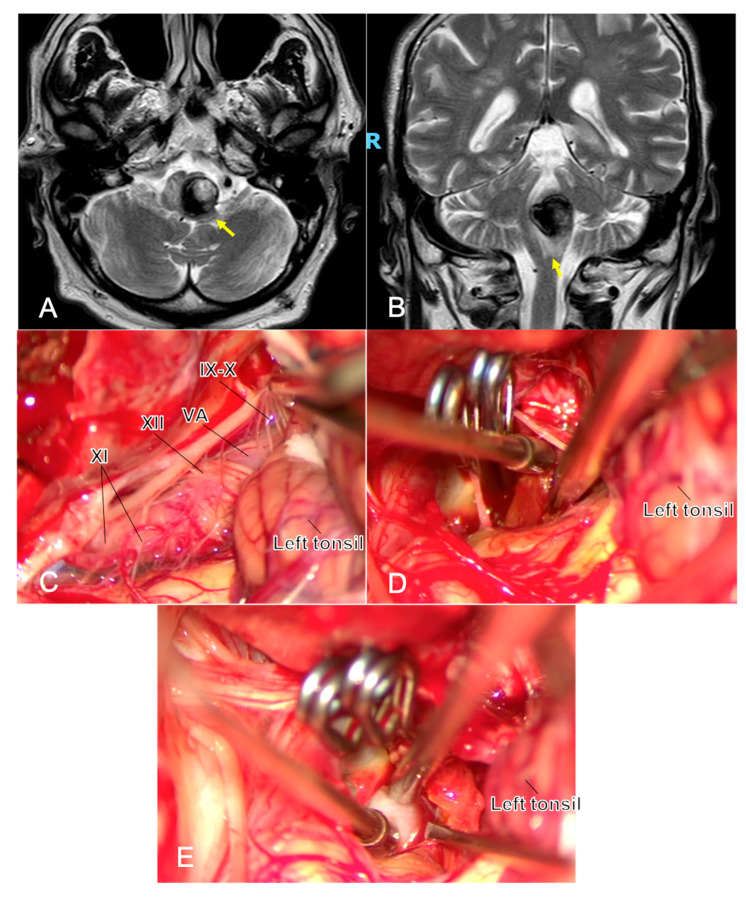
One year after the simple coiling of a previously ruptured p1 PICA aneurysm, a young man developed dysphagia and early right hemiparesis. MRI shows a partially thrombosed recurrent aneurysm at the left VA–PICA junction with marked brainstem compression and surrounding edema ((A,B), yellow arrows). Microsurgical exposure (C) highlights the challenging anatomy at the VA–PICA origin, with the neck arising in close relationship to the lower cranial nerves (IX, X, XI, and XII cranial nerves) and P1 perforators, underscoring the technical difficulty of clip reconstruction in this region. Complete exclusion of the sac is achieved, and the intrasaccular thrombus is evacuated to reduce mass effect (D). Throughout the procedure, micro-Doppler flowmetry and neuromonitoring represent two fundamental tools during this clipping, and in (E), the application of the micro-Doppler flowmetry is shown to confirm proximal/distal vessel patency and complete aneurysm obliteration. *Abbreviations: MRI—magnetic resonance imaging; PICA, postero-inferior cerebellar artery; VA, vertebral artery*.
